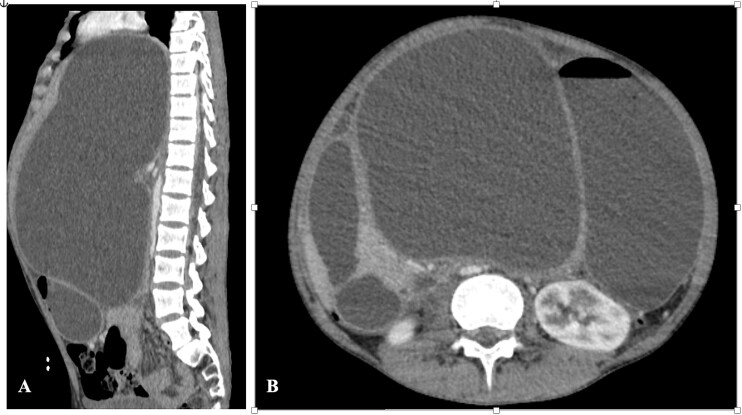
CT abdomen—(A) sagittal section and (B) axial section showing the pseudocyst with significant organ compression.
